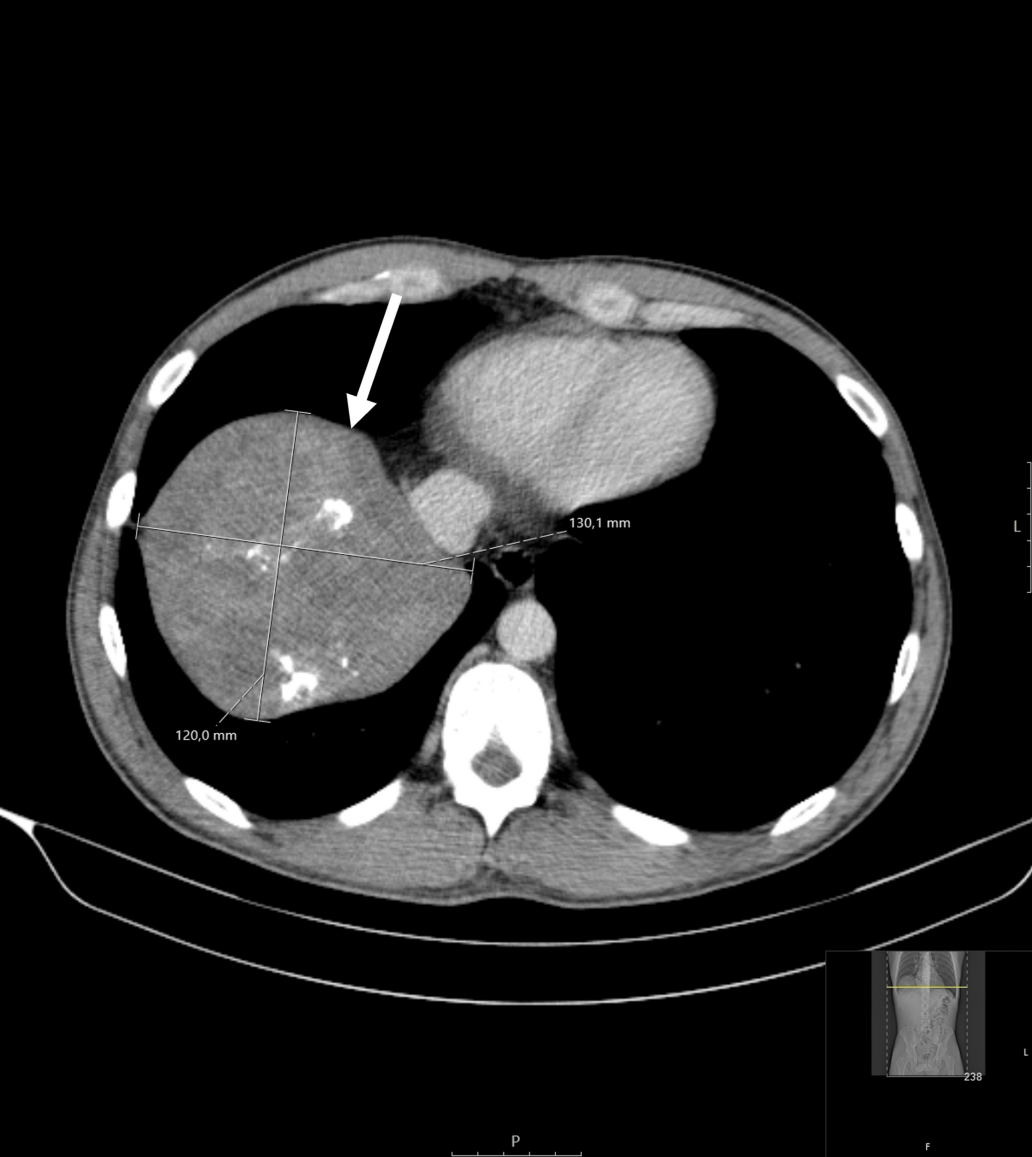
Chest, abdomen, and pelvic computed tomography (CT): axial plane Hypodense mass extending into the thoracic cavity, with areas of calcification, measuring 12 x 13 cm
